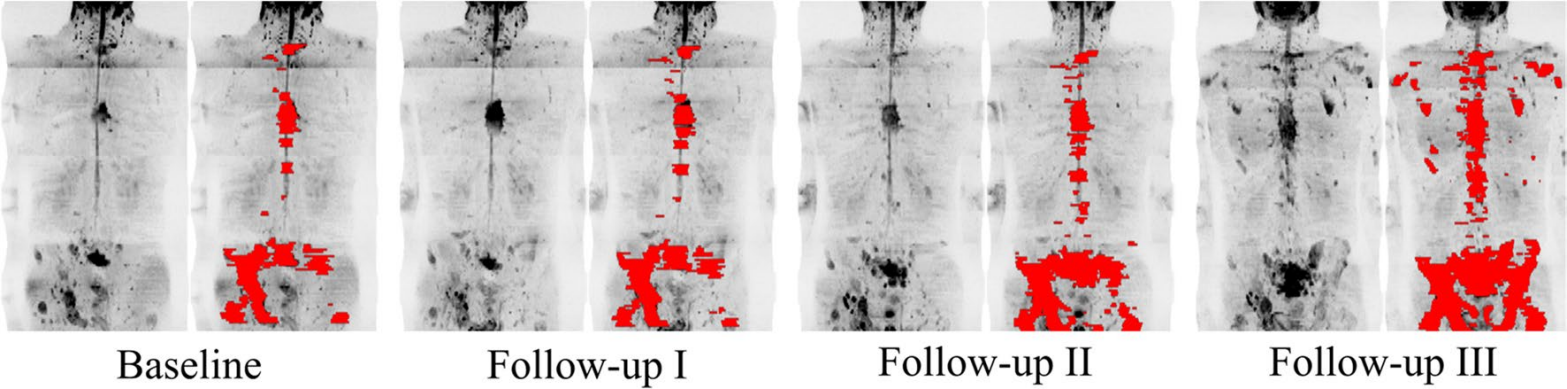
Segmentation of total skeletal disease volume in a 74-year-old metastatic castrate-resistant, non-responding prostate cancer patient on whole-body DWI, maximum intensity projection of the composed *b*900 DWI images with and without superimposed total diffusion volume (red) representing the segmented skeletal disease burden for baseline and three follow-up imaging time-points (I–III)
